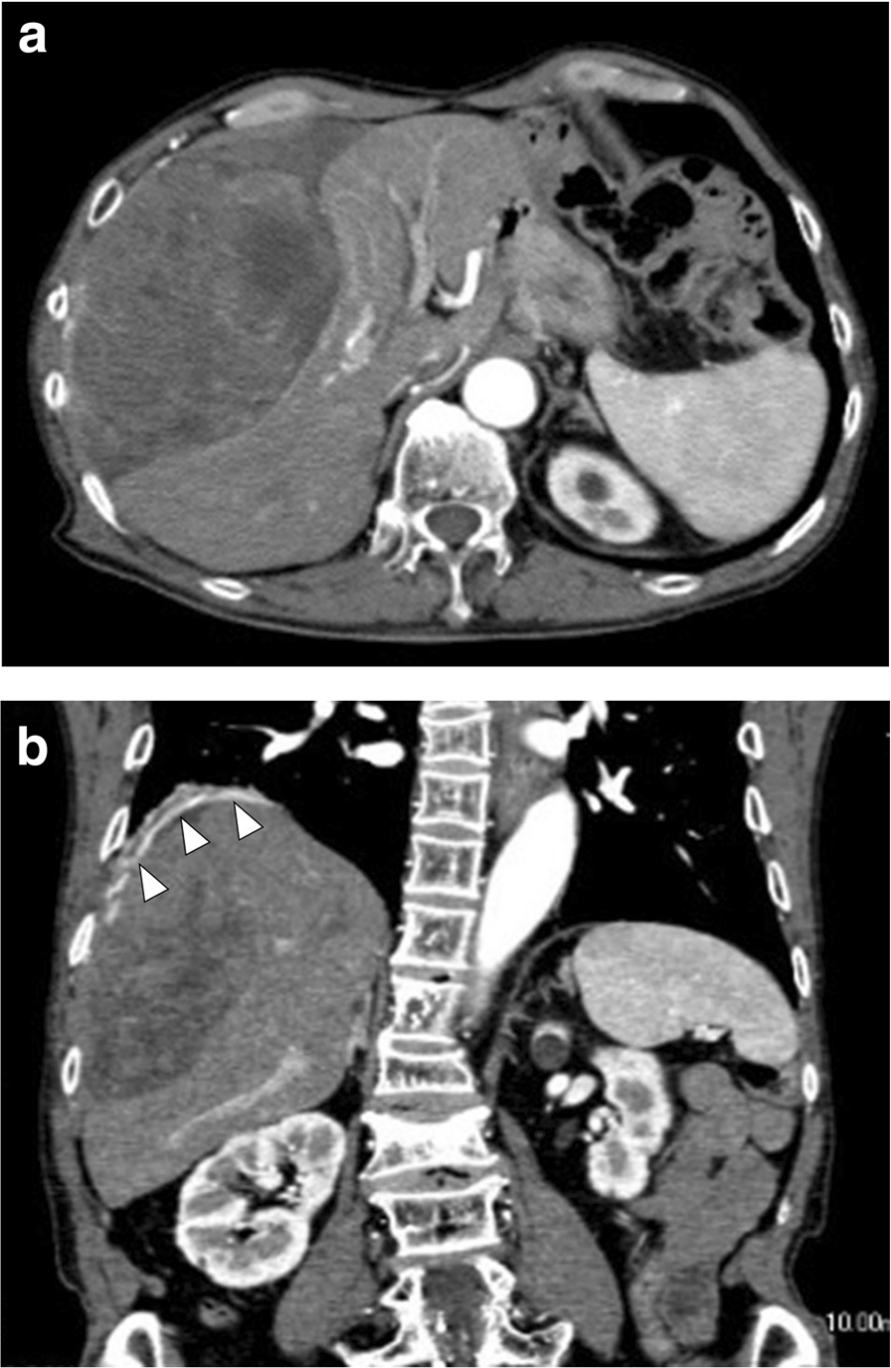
Computed tomography image showing a large subphrenic tumor. The heterogeneously enhanced tumor was well demarcated from the liver (a). The feeding artery originated from the diaphragm (arrowhead) (b)
